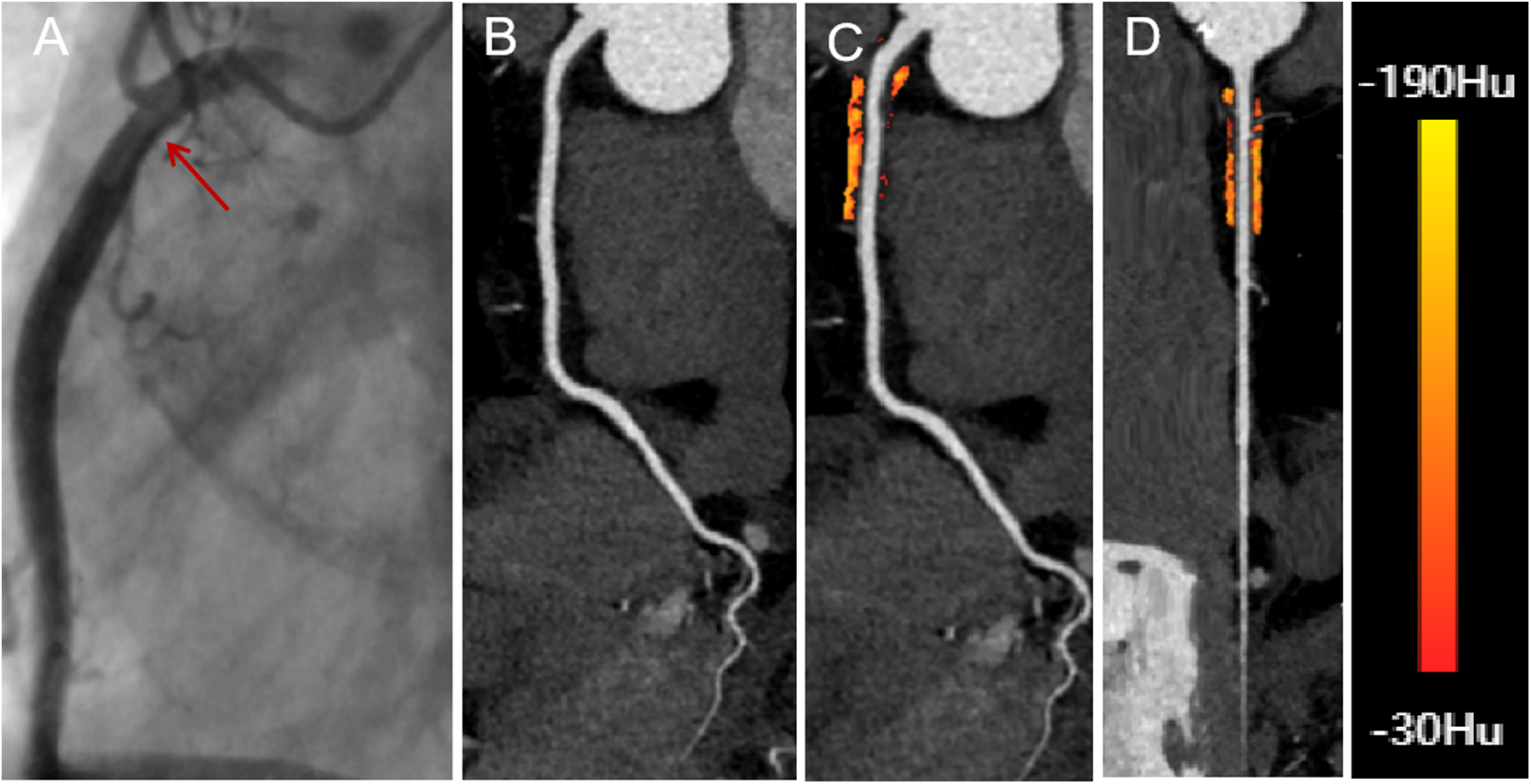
A 66-year-old male with insignificant hemodynamic stenosis in the proximal segment of the right coronary artery. (A) The red arrow shows a narrowing of 30% in the DSA. (B) CCTA with curved planar reformation of the right coronary artery shows non-calcified plaque formation in the proximal segment, with an approximate lumen narrowing of 15%. (C) A representative curved planar reformation image reveals a FAI [−81 HU] of PCAT, suggesting non-ischemic coronary artery narrowing. (D) FAI color maps of the PCAT in the proximal 10–50 mm of the right coronary artery, with a probe straightening image. PCAT color map ranges from red [−30 HU] to yellow [−190 HU]. CCTA, coronary computed tomography angiography; DSA, digital subtraction angiography; FAI, fat attenuation index; PCAT, pericoronary adipose tissue.
